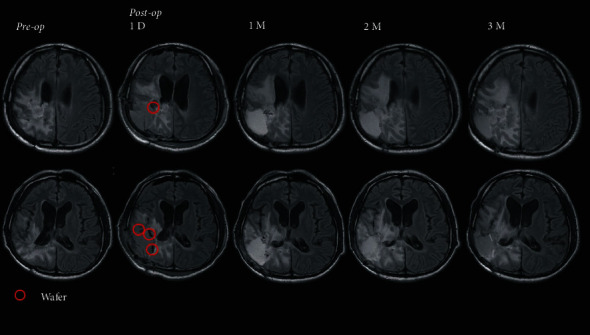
Clinical T2-FLAIR MRI showing brain edema surrounding the right parietal surgical site after intracranial implantation of Cerebraca Wafers. Axial MR T2 FLAIR demonstrating brain edema on the right parietal region. After CWs were implanted (red circles) at suspected residual tumors, stability of brain edema in the 3-month follow-up period, and ventricle size could be observed without significant changes (lower panels).
